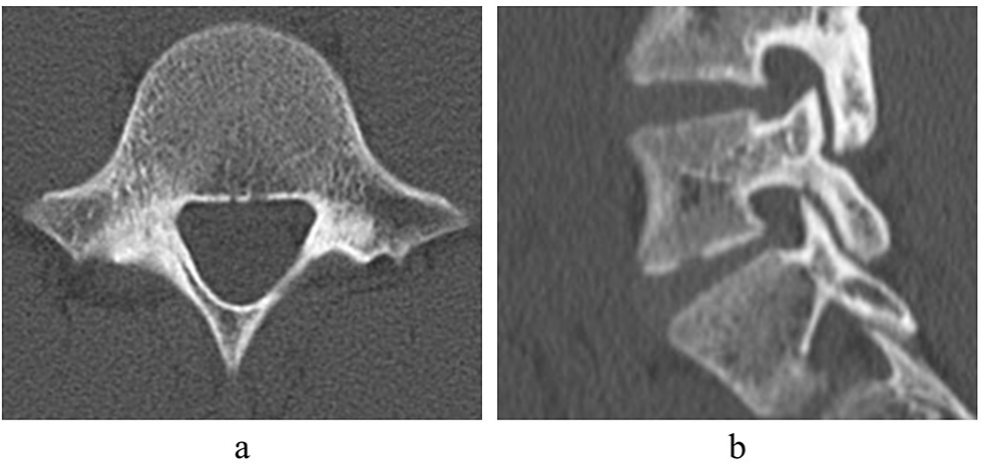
Computed tomography at first examination Computed tomography (CT) showed no fracture line in axial slice and slight bone resorption on the ventral side of the lamina in sagittal slice (a, b).
